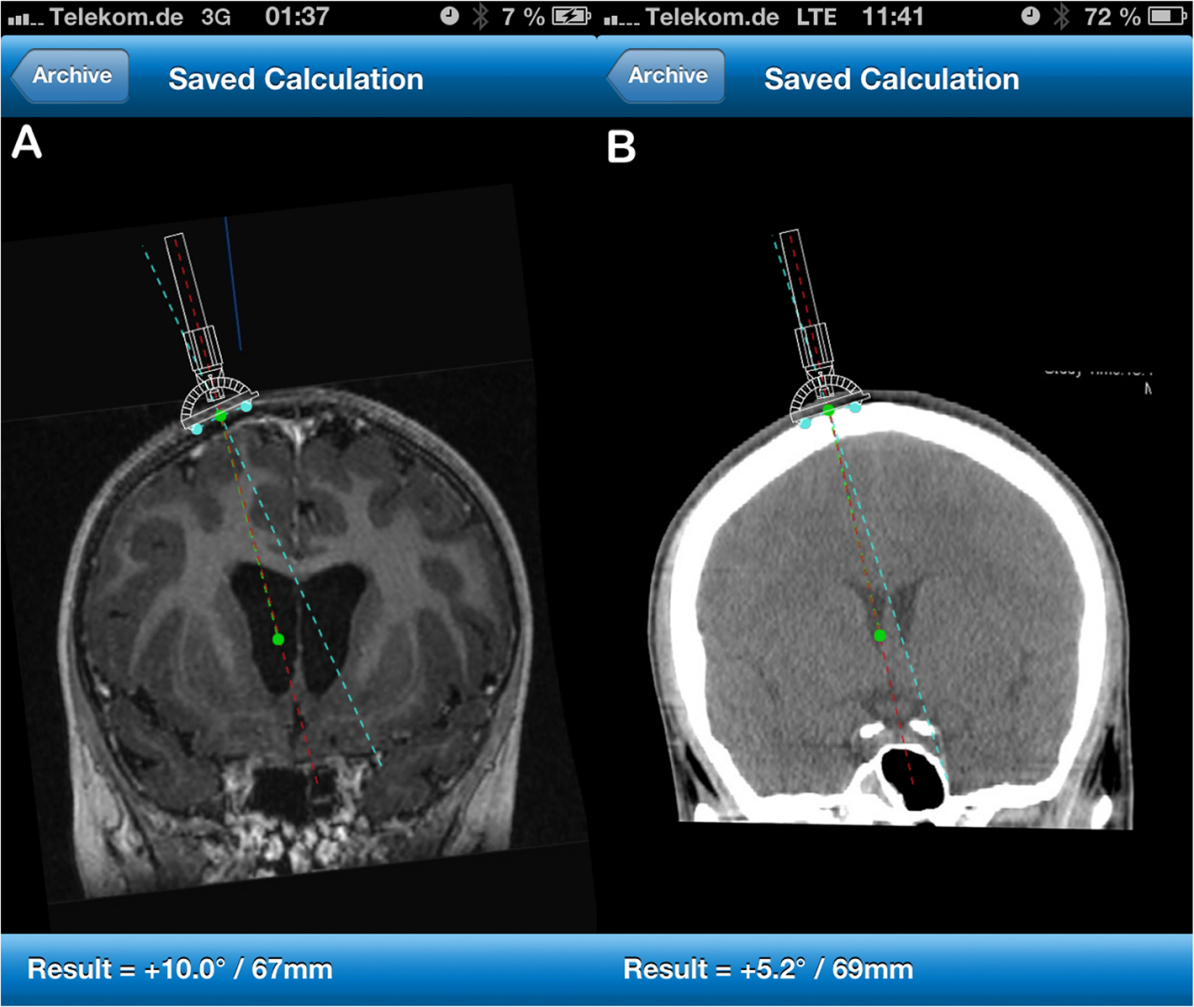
Measurements acquired through the smartphone application. A: Coronal MRI section with moderate enlarged ventricles with virtual placement of the guide on the skull surface. Angulation of catheter trajectory (red dotted line) is measured at 10° deviation from 90° (light blue dotted line) tilting the guiding tube towards the midline. The catheter length (green dotted line) is measured at 67 mm. B: Coronal CT reconstruction along body axis with the measurements revealing an angulation of 5.2° deviation from rectangular with the guiding tube tilt towards the midline and a catheter length of 69 mm.
